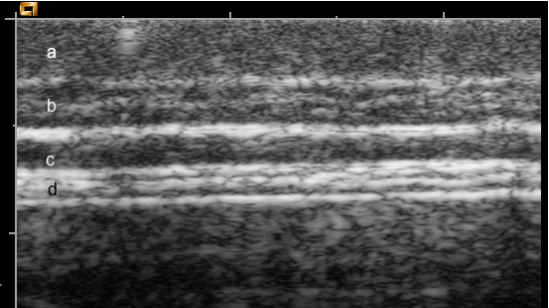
Ultrasound image. This image was obtained during the second scanning session. Layers are marked as follows. (a) skin, (b) outer, (c) middle and (d) inner, adipose layer.
